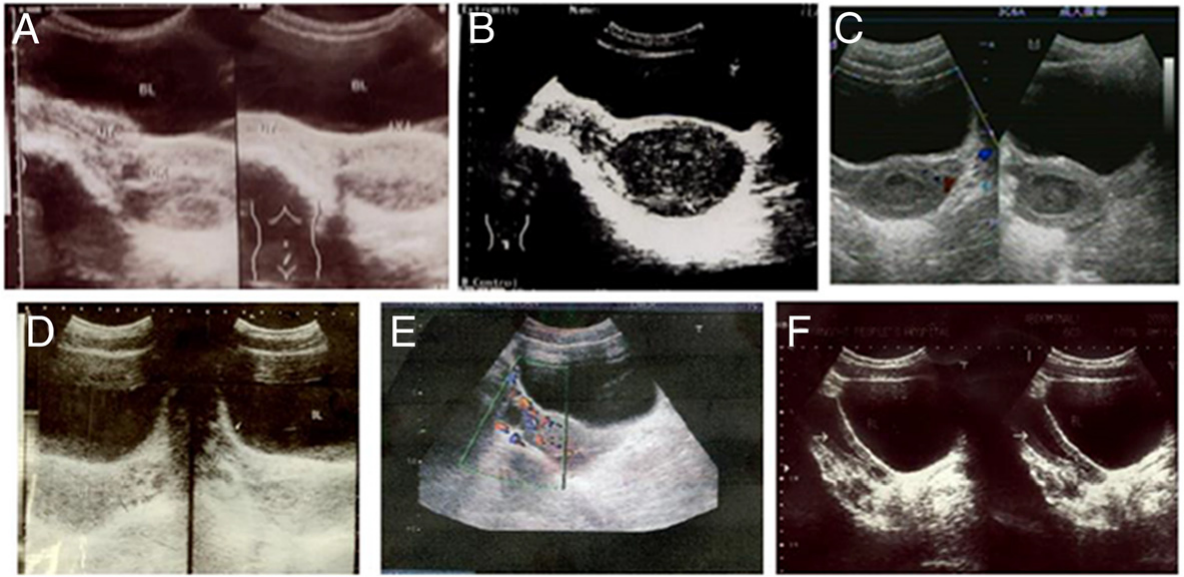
Characteristics of congenital cervical atresia in preoperative ultrasonography. (A, B) Type I congenital cervical atresia, showing the unique shape of a Type 1 uterus and uterine isthmus. (C) Type II congenital cervical, atresia showing a hematocele in the uterine cavity and a thickened lower uterine cavity (blind end) and wall. (D) Type III congenital cervical atresia, showing a sinus-like cervix becoming narrower from the top to the bottom; the end of the cervix was significantly enlarged. (E) Type III congenital cervical atresia, showing a long and solid cervix, with no enlarged end. (F) Type IV congenital cervical atresia, showing thickening of the wall of the cervical canal and a blind end. The liquid anechoic area of the uterine cavity was bigger than that of the cervix.
